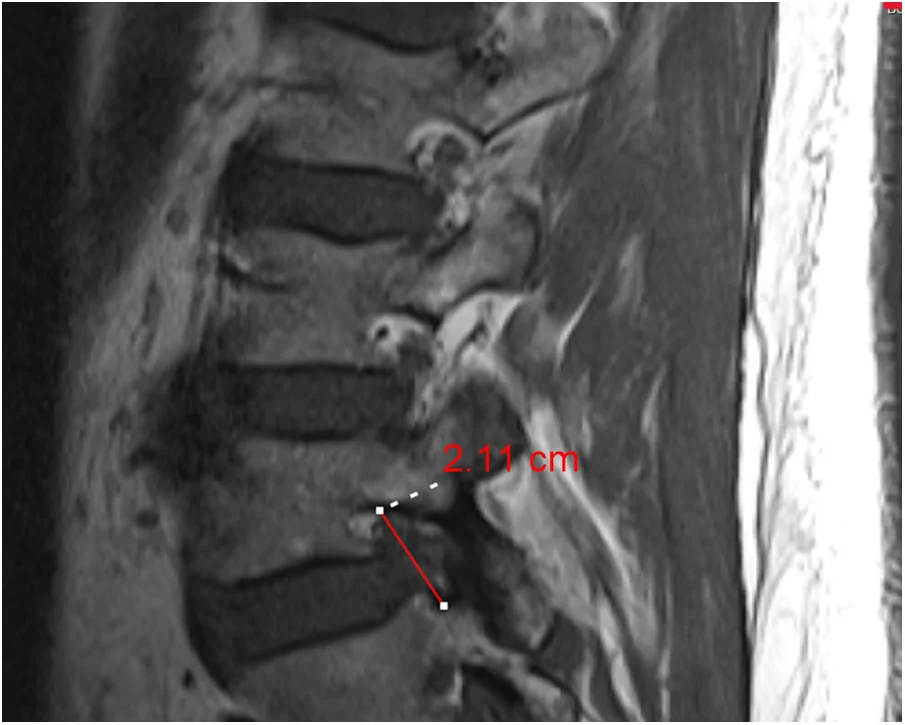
Magnetic resonance imaging (MRI) example measurement of foraminal height.
